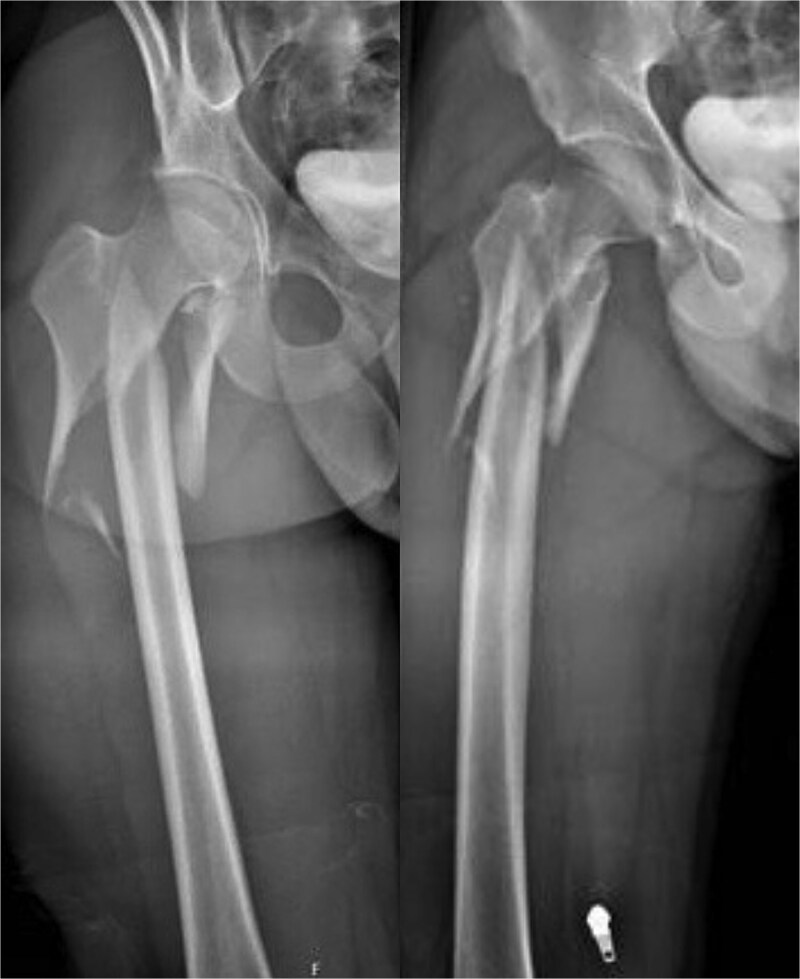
Pre-operative anteroposterior (left) and lateral (right) radiographs of the injured femur.
